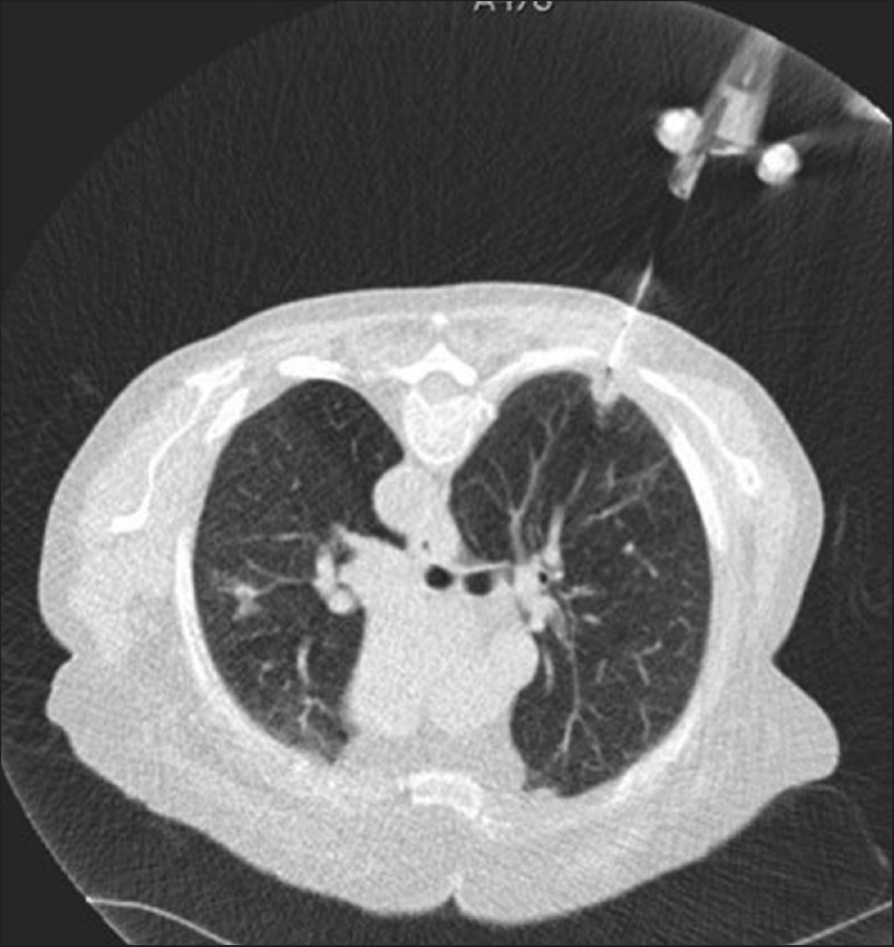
CT fluoroscopic image during a CT fluoroscopy guided transthoracic biopsy of a subpleural pulmonary lesion anteriorly in the left upper lobe.
